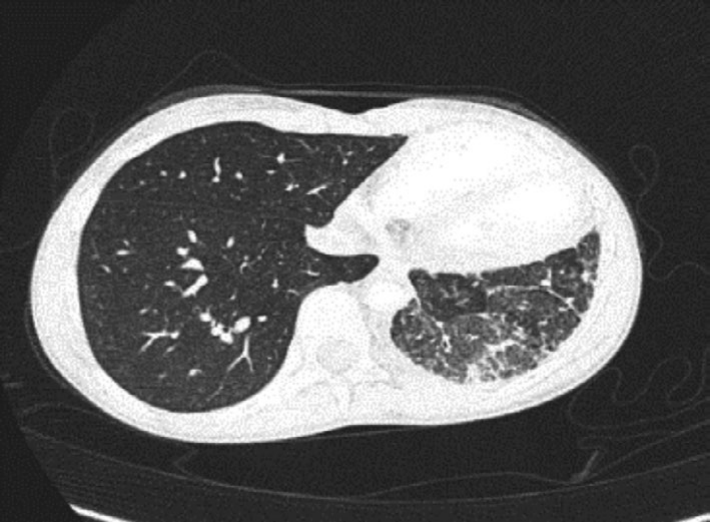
CTA—abnormalities in lung parenchyma and vasculature. The pulmonary veins were not demonstrated near the LA
